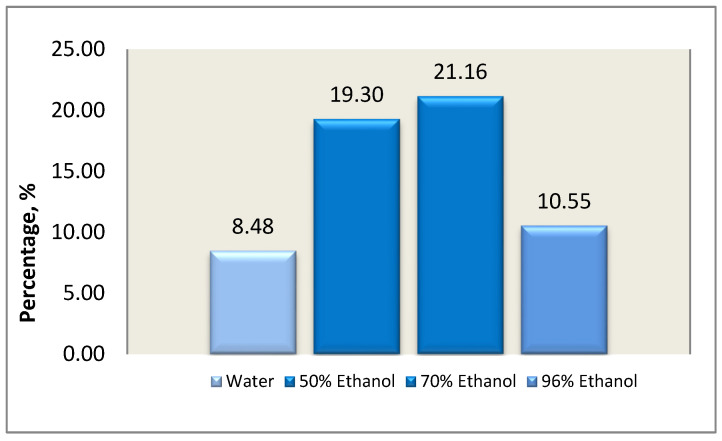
Extraction yield isolated from *I. scariosa* plants during ultrasonic extraction using ethyl alcohol of varying concentrations.
